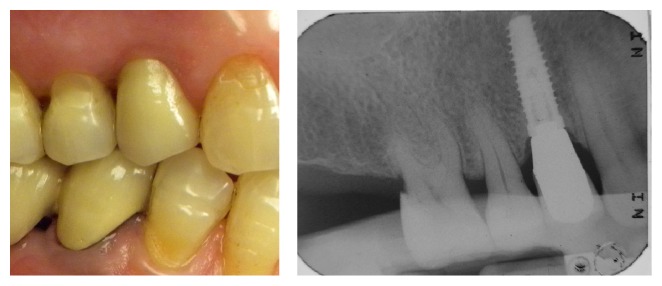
Case 2: 6 years of follow-up and radiographic control.
